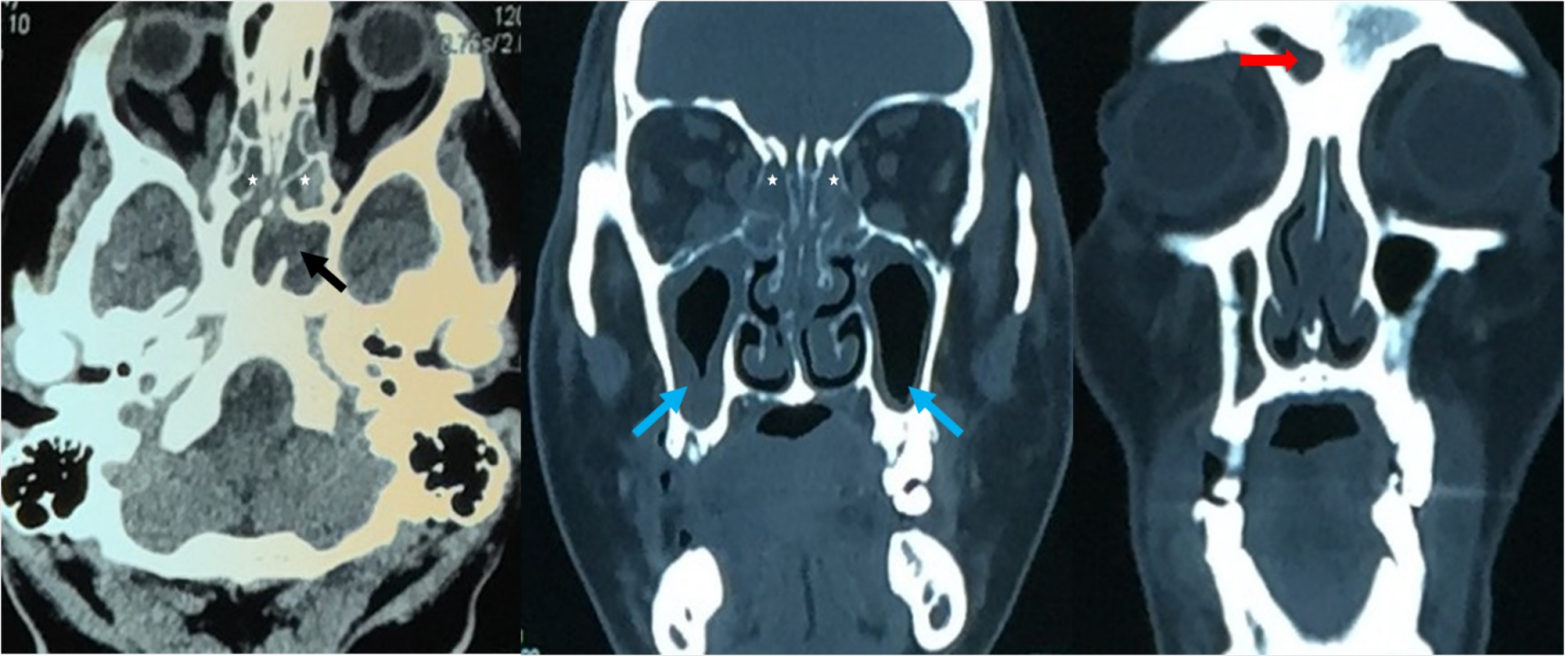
Non-contrasted CT on initial presentation showed mucosal thickening in ethmoid sinus [white star on axial and coronal plane], sphenoid sinus [black arrow on axial plane], maxillary sinus [blue arrow on coronal plane] and frontal sinus [red arrow on coronal plane] indicating pansinusitis CT: computed tomography
